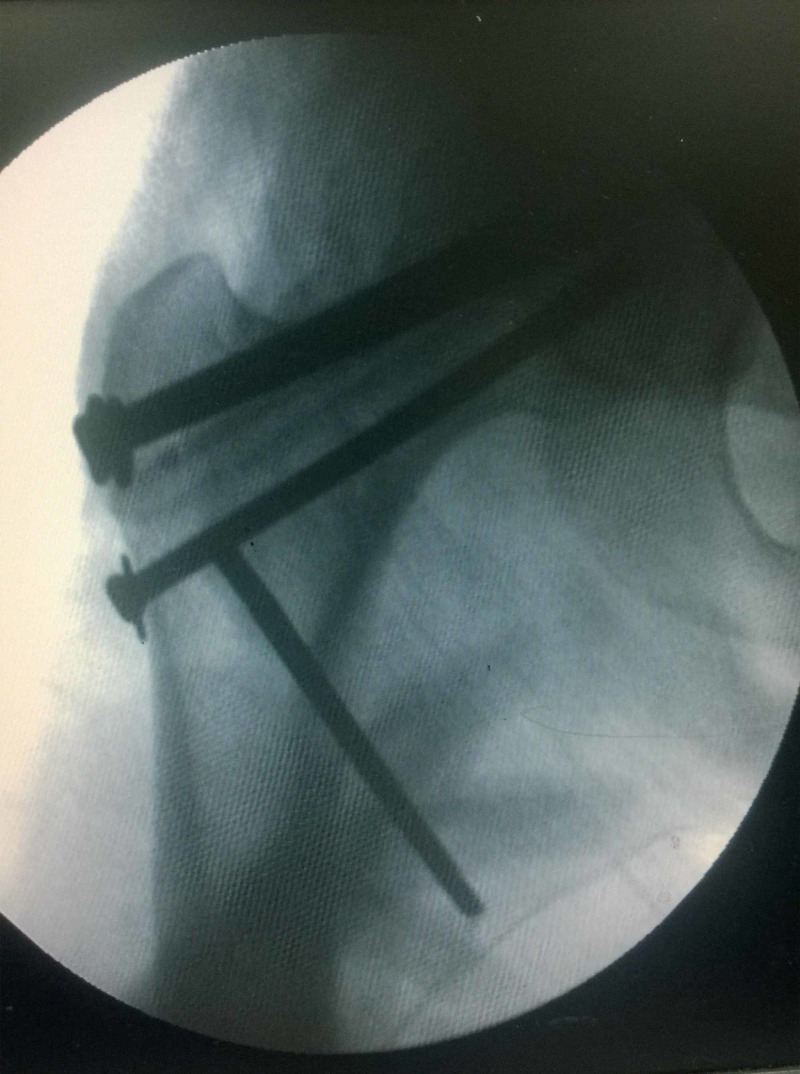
Intra-operative radiograph of patient A showing the three screws and the central fibula. The Schanz screw at the level of lesser trochanter was used to control rotation during reduction.
